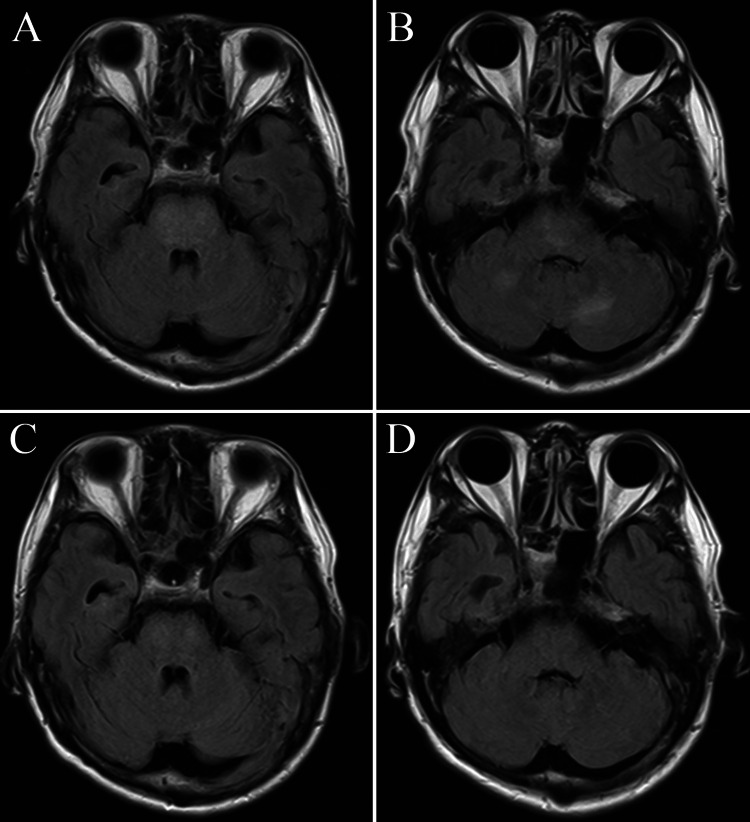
Magnetic resonance imaging of the head during hospitalization (A,B) Cranial magnetic resonance imaging on day 4 of admission. Fluid-attenuated inversion recovery (FLAIR) images show lightening of the high signal in the pons and cerebellum. (C,D) Cranial magnetic resonance imaging on day 21 of admission. On FLAIR images, the high-signal lesions have disappeared.
